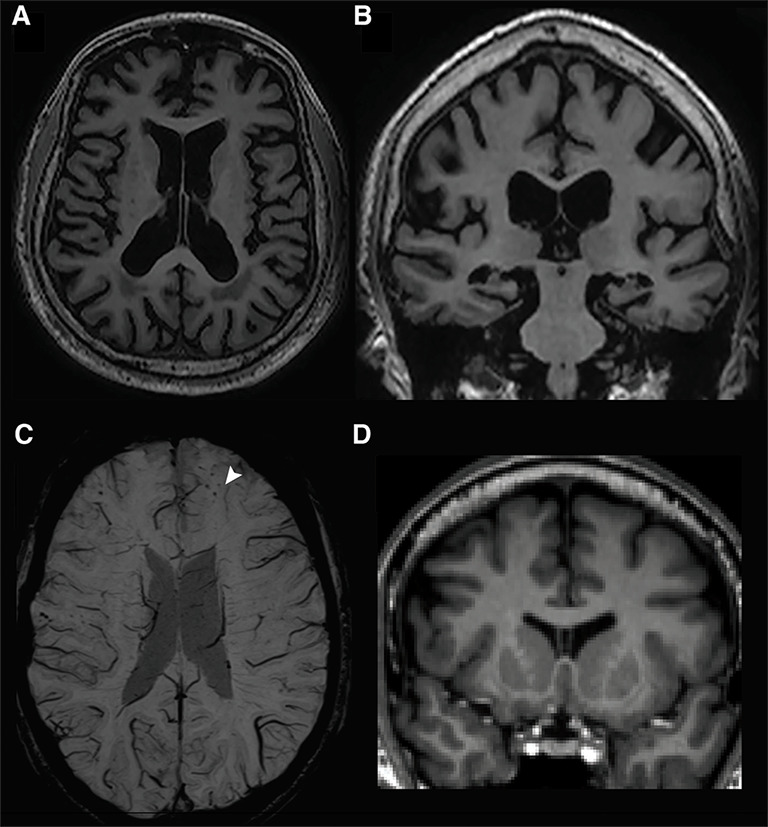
Neuroimaging features in former contact sports athletes. Volumetric T1 MR scan of brain in a patient in their 80s with history of American football participation and clinical syndrome of dementia, with CTE present at post-mortem. Axial (A) and coronal (B) views showing prominent frontotemporal atrophy and cavum septum pellucidum posteriorly; images adapted with permission from Alosco *et al.*^39^ (C) Axial SWI MRI in midlife former rugby player showing left frontal microbleeds (white arrowhead). (D) Cavum septum pellucidum shown on coronal T1 MRI in former rugby player. CTE, chronic traumatic encephalopathy; SWI, susceptibility-weighted imaging.
